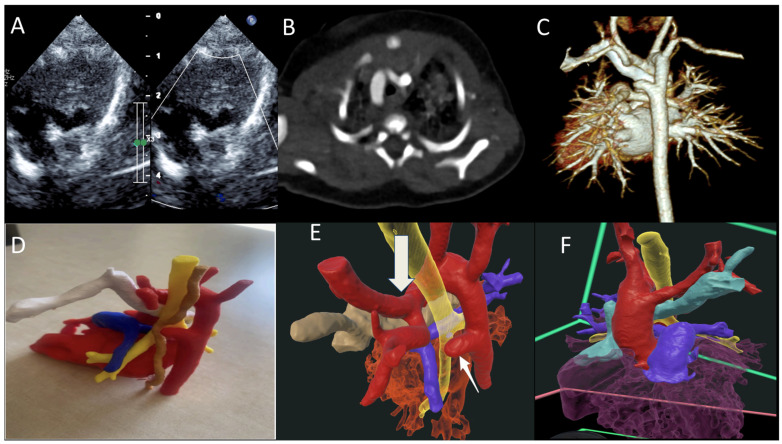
(A) TTE showing double aortic arch with dominant right arch arrow (DAA); (B,C) CT scan of the DAA with dominant right aortic arch, Kommerell diverticulum and atretic left arch; (D) 3D-printed model trachea (yellow), esophagus (brown), retro-aortic innominate vein (white); (E,F) VR showing DAA (red), trachea (yellow), retro-aortic innominate vein (teal) ring, completed by the atretic left aortic arch, Kommerell diverticulum (arrow).
